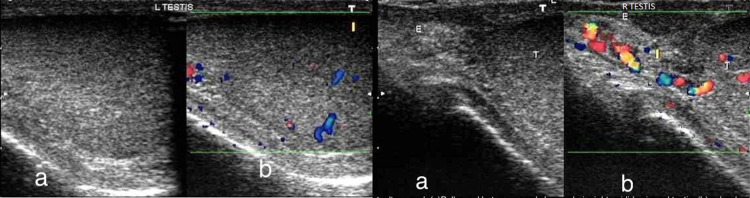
Ultrasound Left testis (a) Greyscale image showing hypoechoic echo pattern of left testis and (b) Color Doppler demonstrating increased vascularity. Ultrasound Right testis (a) Bulky and heterogeneously hypoechoic right testis and epididymis and (b) Color Doppler demonstrating increased vascularity in both structures.
